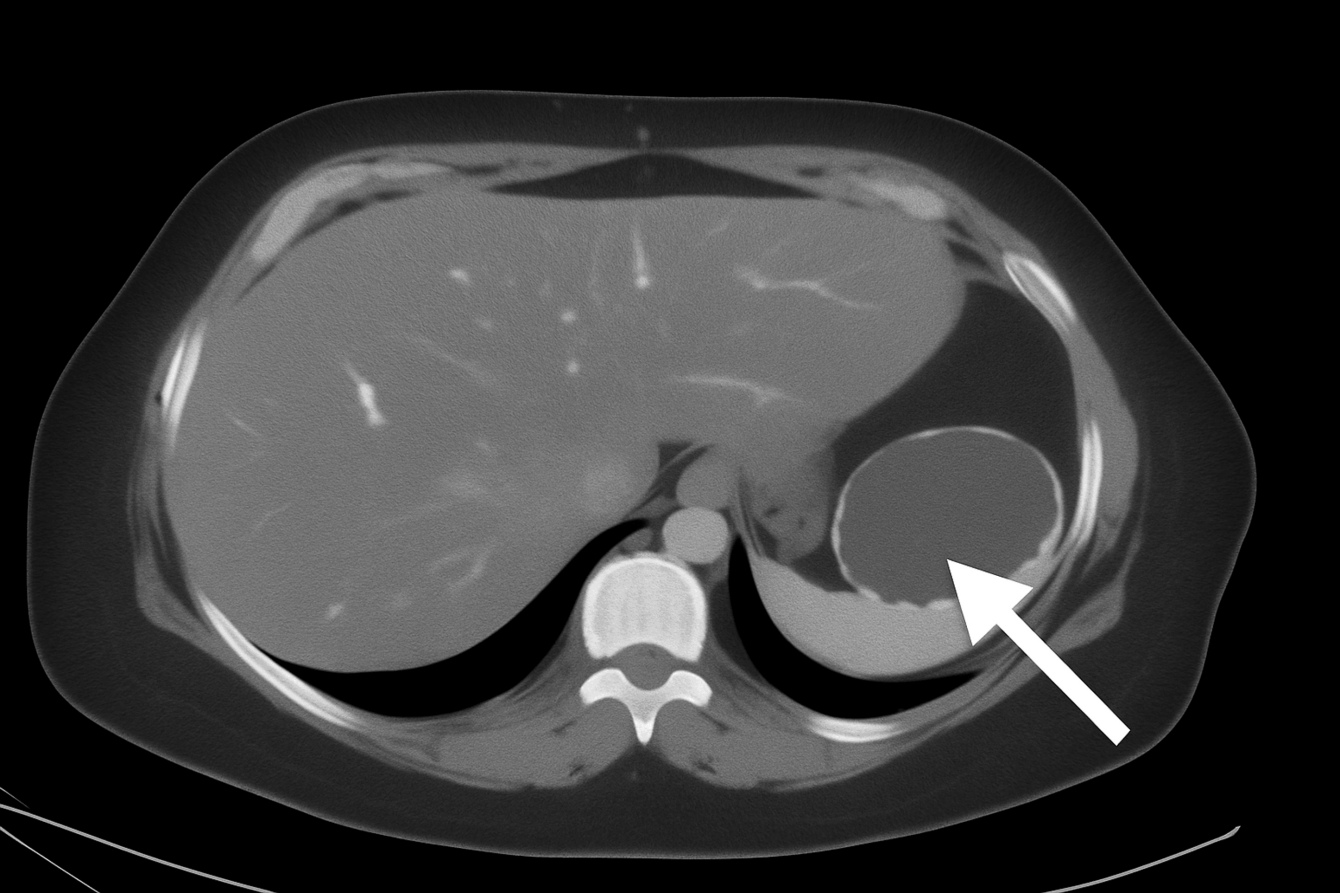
Axial contrast-enhanced CT scan demonstrating a splenic epithelial cyst A well-defined, unilocular cystic lesion is seen in the upper pole of the spleen (white arrow), measuring approximately 6–7 cm, with no internal septations or solid components. Findings are consistent with a benign epithelial cyst.
